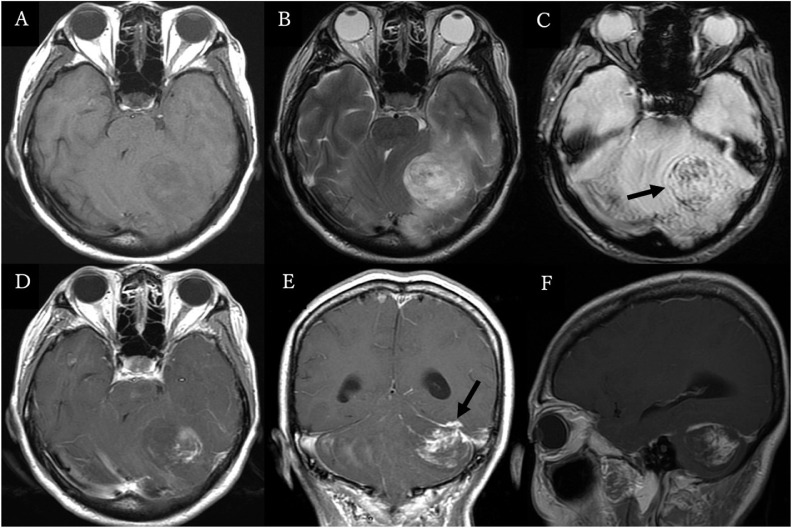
Precontrast magnetic resonance (MR) images of the head showing hypointensity on the T1-weighted image (A), hyperintensity on the T2-weighted image (B), and intratumoral bleeding on the heavy T2-weighted image (C, arrow). Postcontrast MR images showing heterogeneous enhancement of the mass attached to the left cerebellar tentorium (D-F). Note that the supratentorial extension of the tumor was clearly demonstrated on E (arrow).
